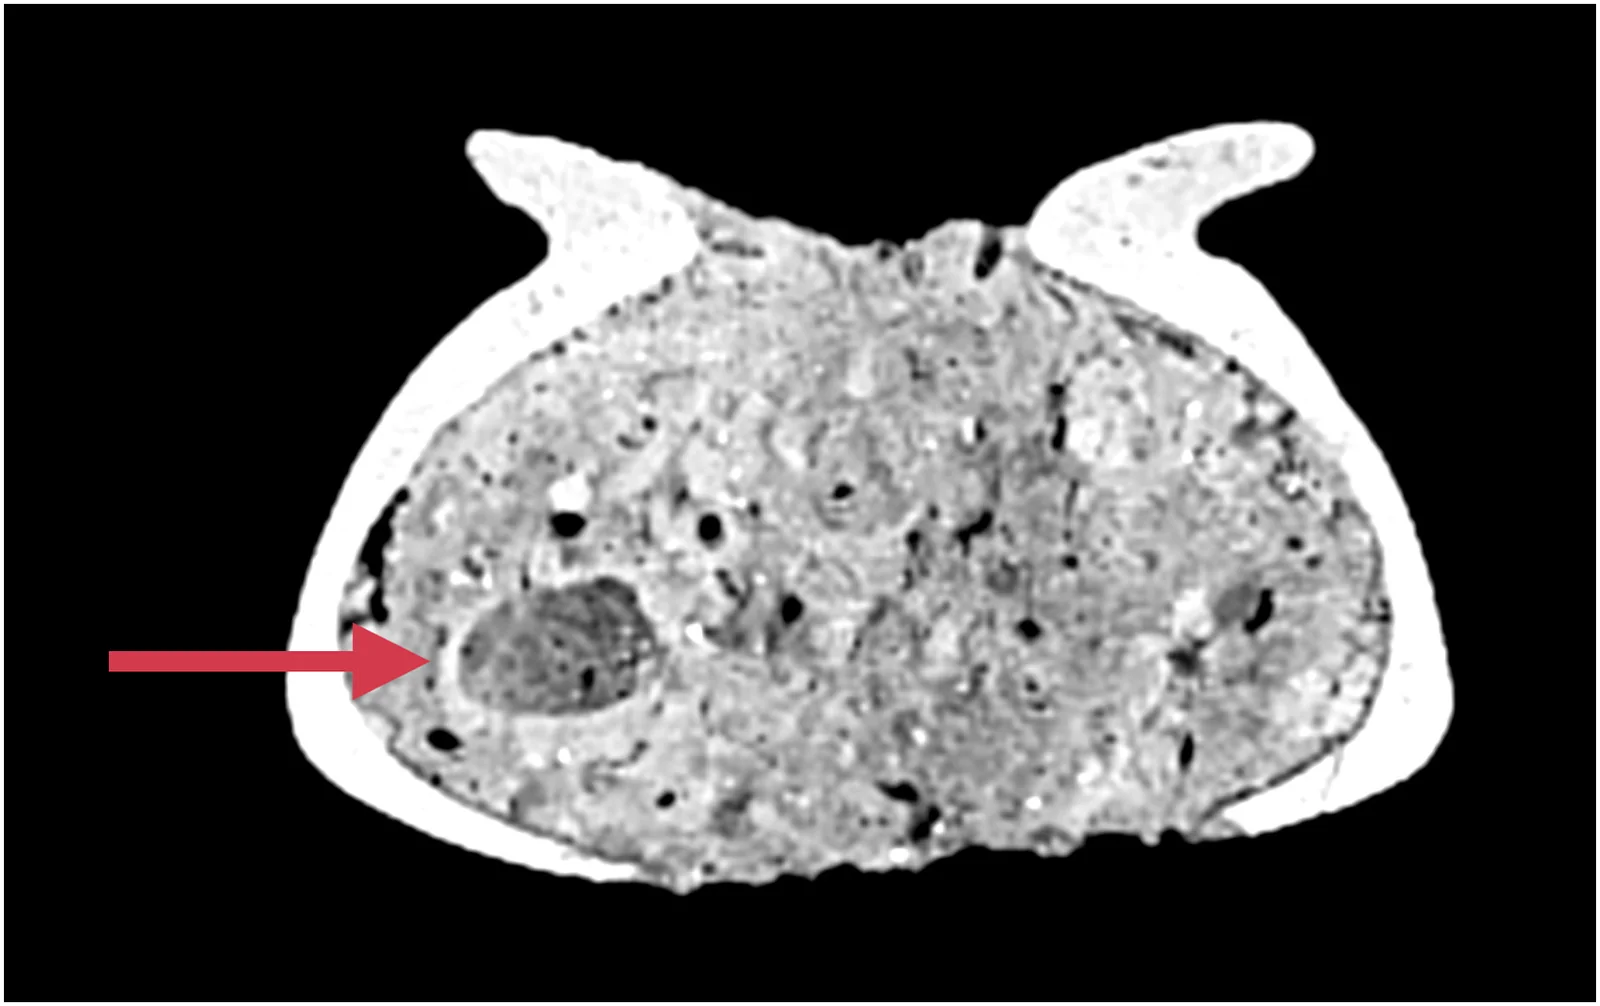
CT-scan examination of sacred Vodun objects (Benin): Cross-vertical-section of ceramic #2 with a negative imprint of a plant offering (cola or palm nut?) (large arrow). Again, the profile and thickness of the entire ceramic, as well as its areas of weakness, appear and are measurable.
